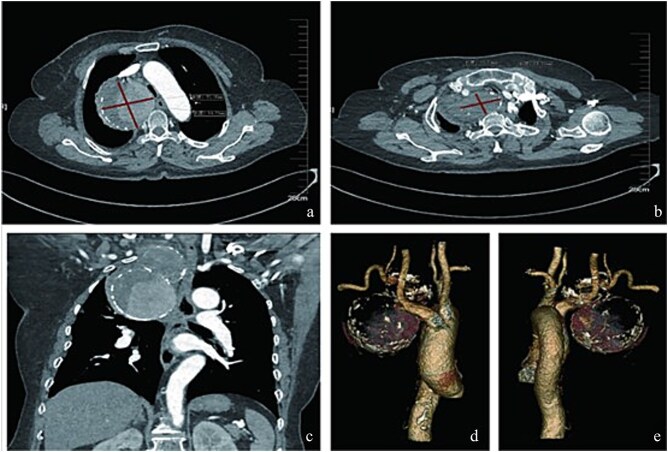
Radiologic characteristics of the SAA. (a) Axial CTA demonstrates a bipartite aneurysm morphology with superior–inferior compartmentalization. The inferior spherical component measures 70.05 mm (long axis) × 69.73 mm (short axis), exhibiting regular contour and homogeneous attenuation. (b) The superior ellipsoid component displays dimensions of 51.81 mm (long axis) × 35.59 mm (short axis), with peripheral thrombus formation. (c) Coronal reformation reveals the characteristic gourd-shaped configuration and intraluminal thrombus burden. (d and e) Volume-rendered reconstructions delineate circumferential calcifications along the aneurysm wall, particularly prominent at the inflow/outflow transitional zone.
